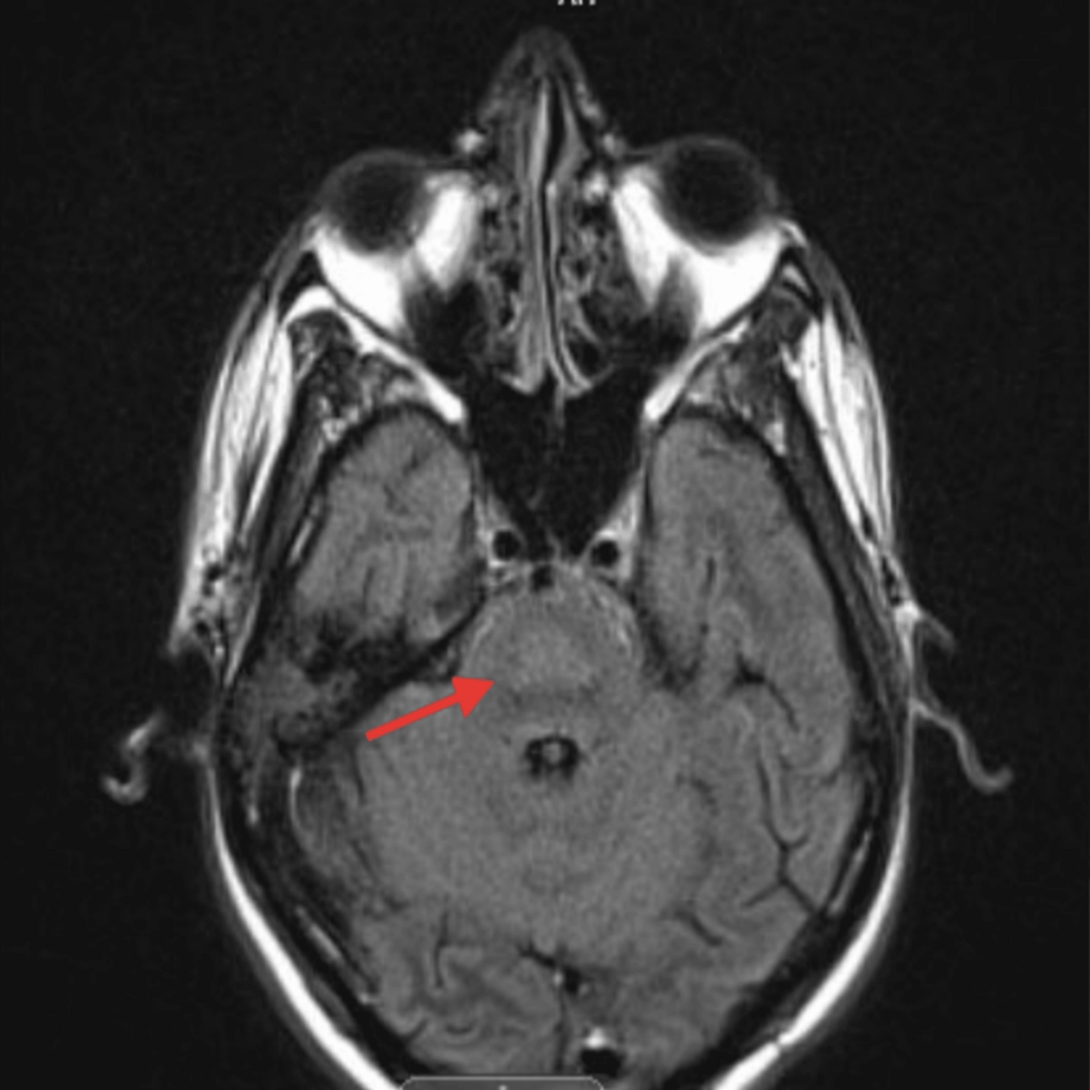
Brain MRI - image 2 The image shows diffusion restriction within the central pons, resembling an incomplete trident sign (arrow) MRI: magnetic resonance imaging
